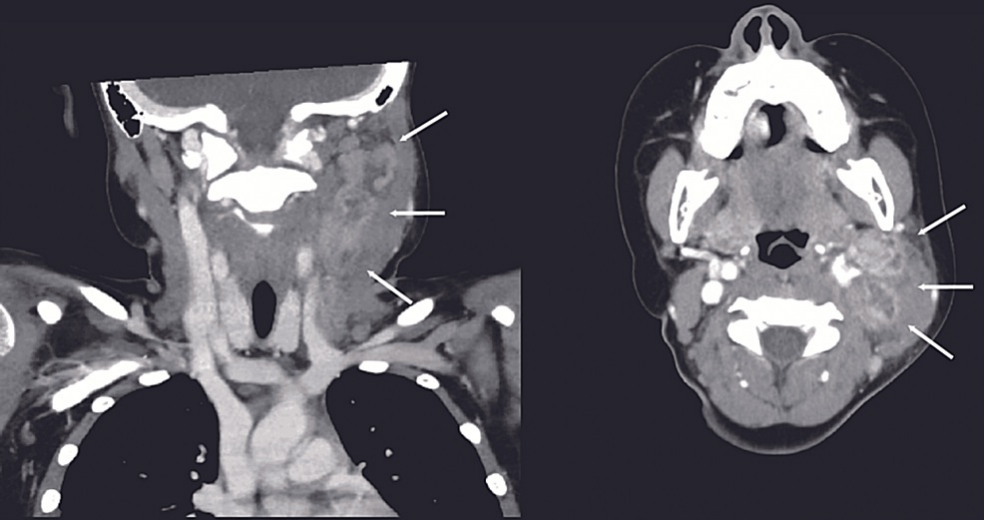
Coronal and axial cuts of a contrast-enhanced computed tomography of the neck Coronal and axial cuts of a contrast-enhanced computed tomography of the neck showing multiple enlarged and enhanced lymph nodes in the left cervical chain (arrows).
